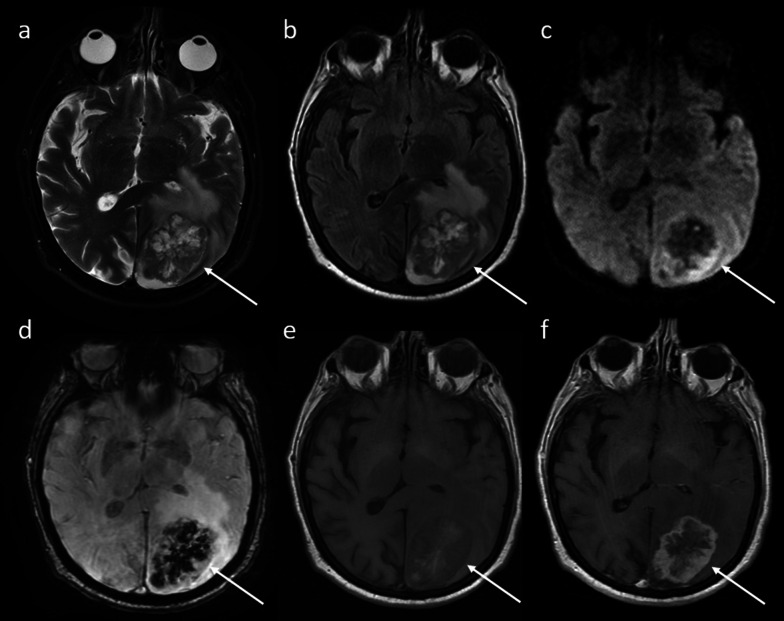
Brain recurrence. Axial MR imaging exhibits a left cerebral lesion (arrows) associated with perilesional edema. The lesion shows inhomogeneous hyperintensity in T2 (a), FLAIR (b), and DWI (c), and hemorrhagic changes in susceptibility-weighted sequence (d) and T1 (e). This brain recurrence of HCC appears as a ring-enhancing lesion in contrast-enhanced MR imaging (f)
